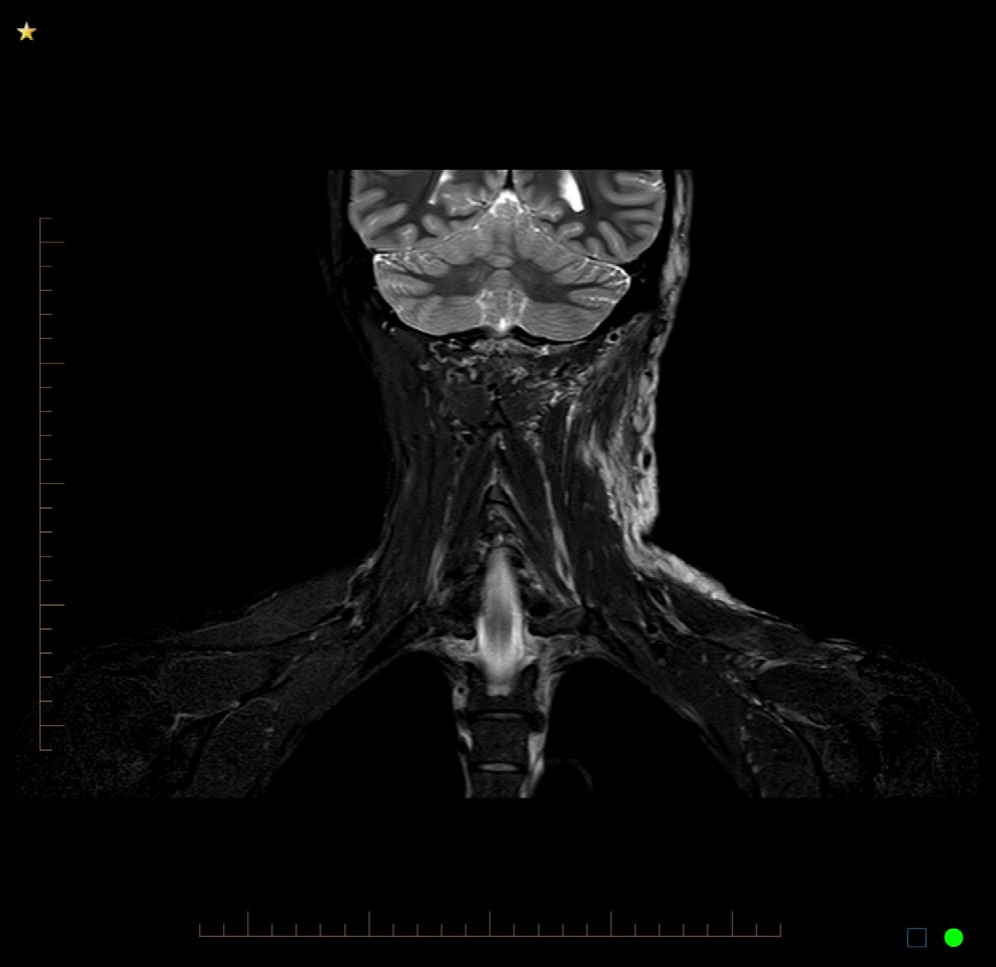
MRI showing T2 hyperintense lesion, more extensive than clinically evident.
